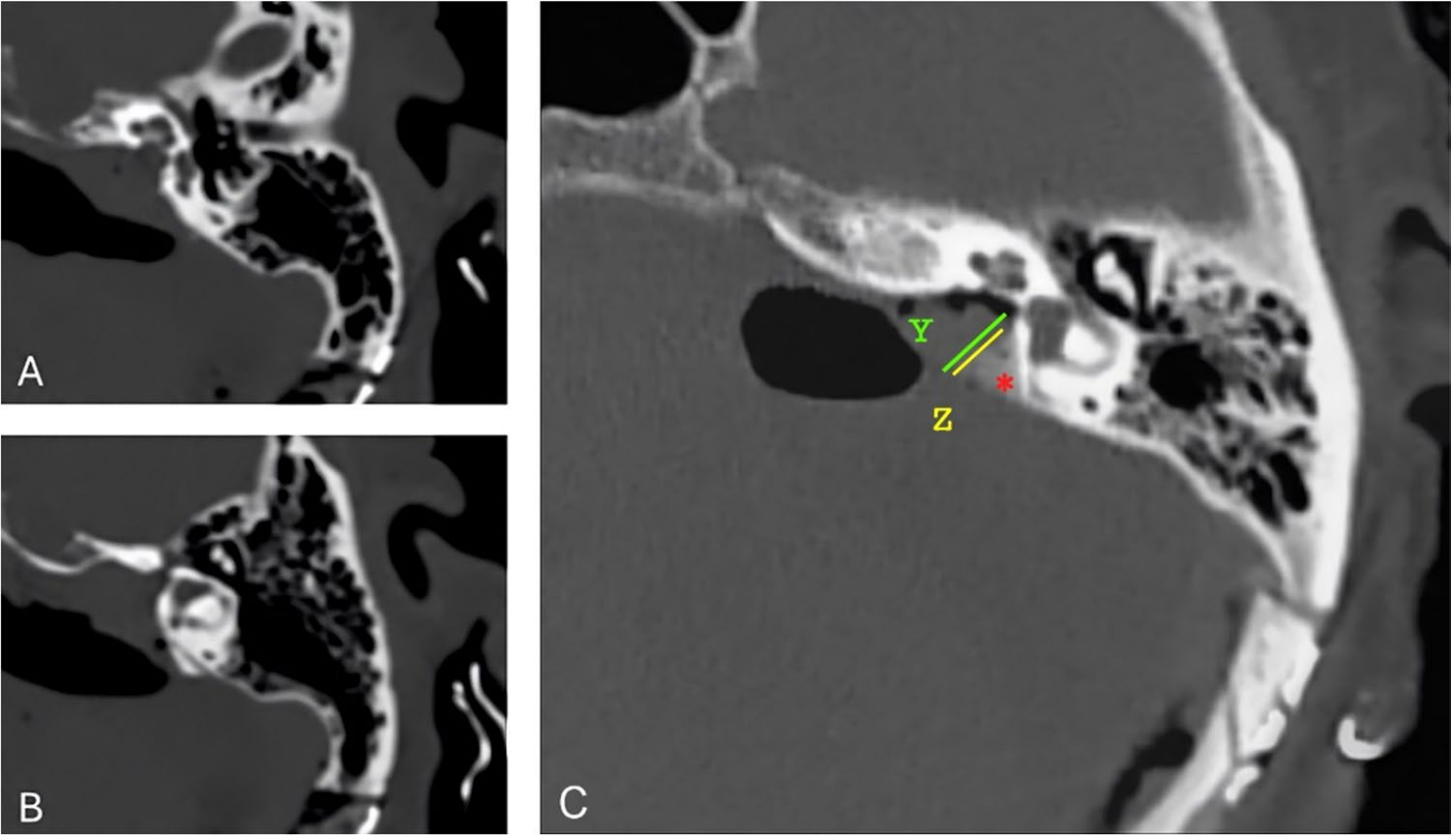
High-resolution CT scan of a patient included in our cohort. A, B Bone-window postoperative HR-CT images used to assess labyrinthine structural integrity. C Pre- and postoperative bone-window HR-CT images merged to measure IAC drilling parameters. Line Z indicates the length of the internal auditory canal drilled. Line Y indicates the total length of the internal auditory canal. The angle of safe neuronavigated drilling is marked with the asterisk
